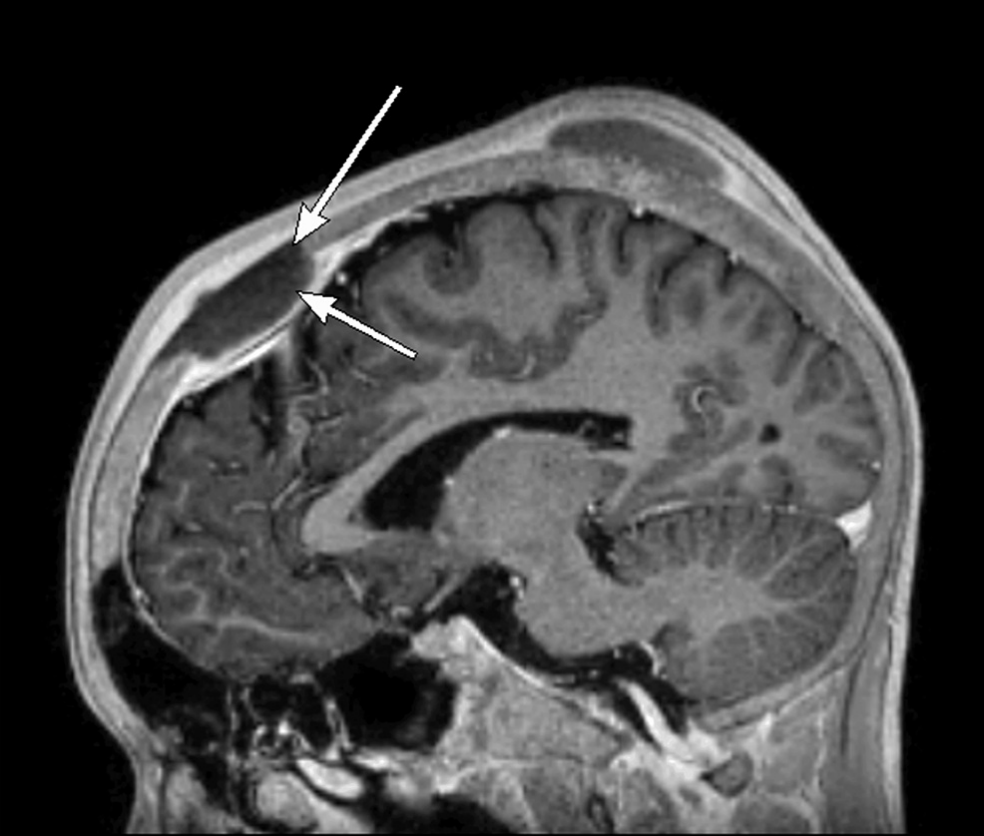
Sagittal MRI with contrast. Post-contrast MRI shows compression and inferior displacement of the superior sagittal sinus secondary to the overlying epidural hematoma. Arrows indicate bone infarction (top arrow) and associated hematoma (bottom arrow).
